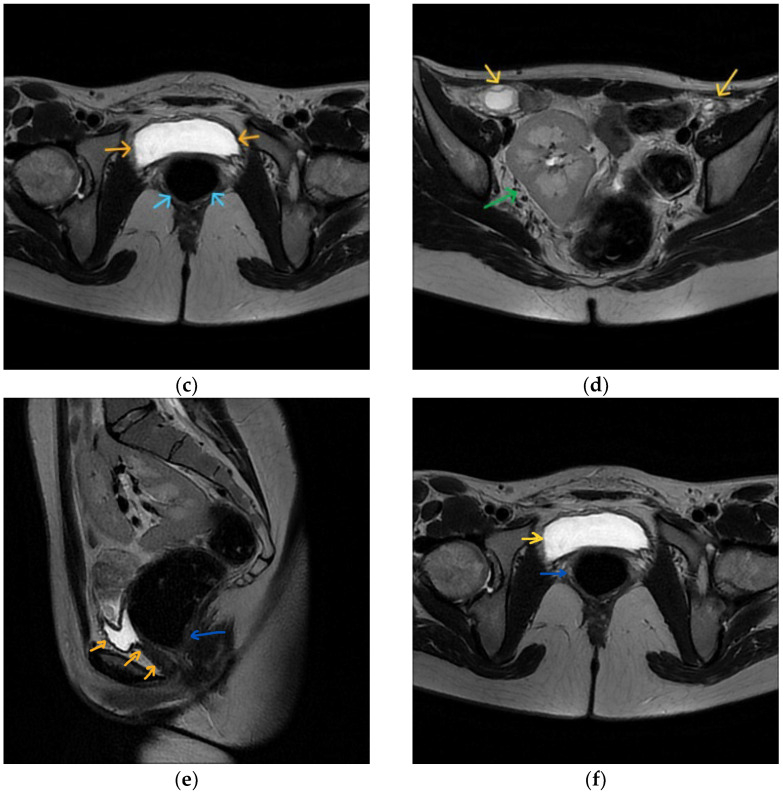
MRI images of the patient, with a series of objectified anomalies, all compatible with MRKH syndrome type 2. Coronal (a,b), axial (c,d,f) and sagittal (e) views. Bladder (orange arrows), rectum (light blue arrows), ovaries (yellow arrows), ectopic kidney (green arrows), hemivertebra (dark blue arrows) are outlined.
